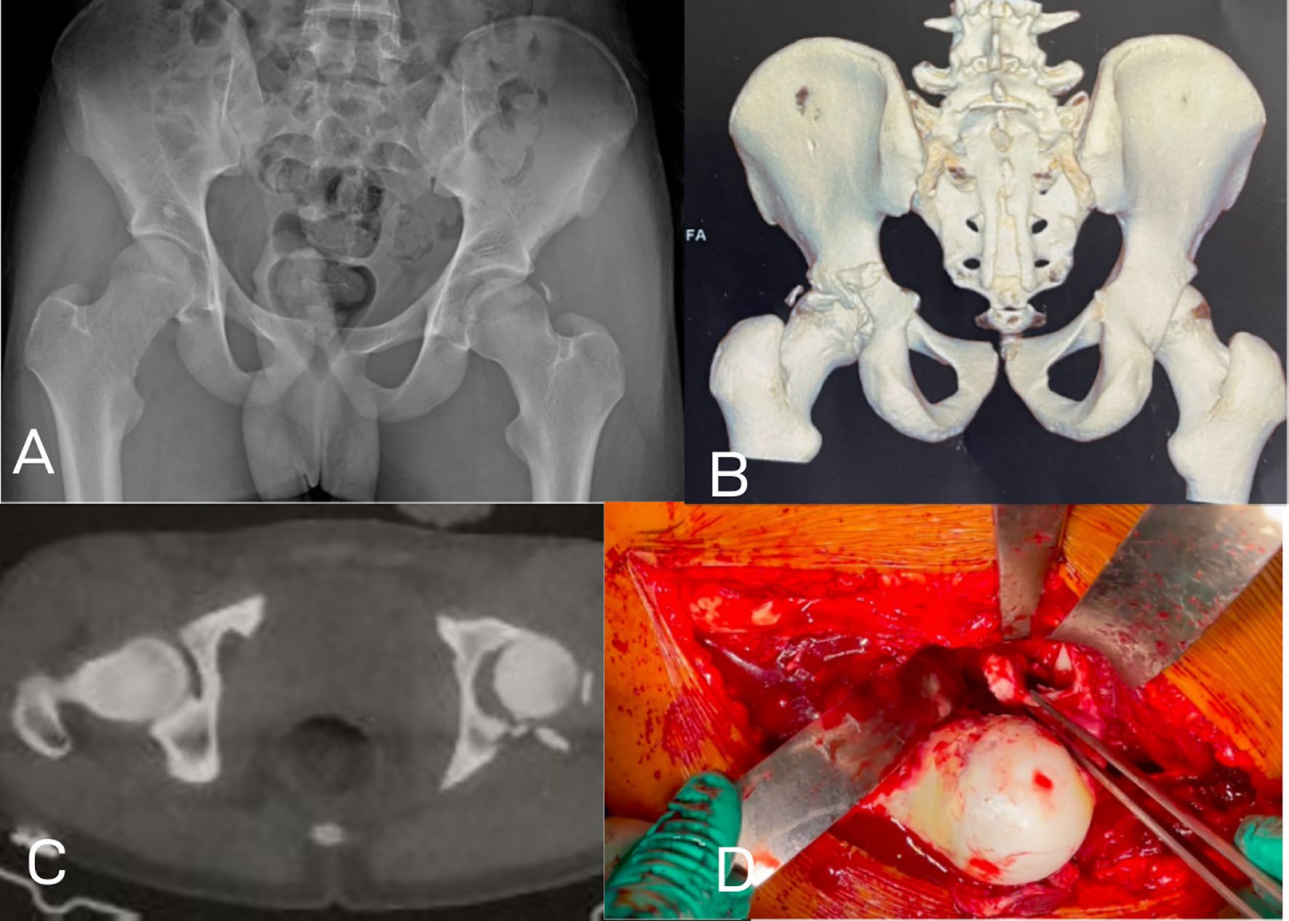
Labral avulsion; A AP radiograph showing sublaxed left hip with tiny posterosuperior bony fragment. B 3D reconstruction and C axial CT cut showing multipl tiny fragments of the posterior wall D intraoperative photo during surgical hip dislocation showing the lesion to be avulsion the posterior labrum with small bony rim attached
